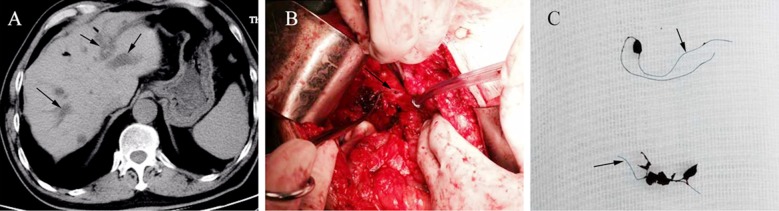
Case 2: A. CT scan, showing dilation of the intrahepatic bile duct (black arrows). B. The stone was found after the anastomosis (black arrow) was opened. C. Several bile duct stonesformed around the Prolene sutures. The black arrows indicate the Prolene sutures.
